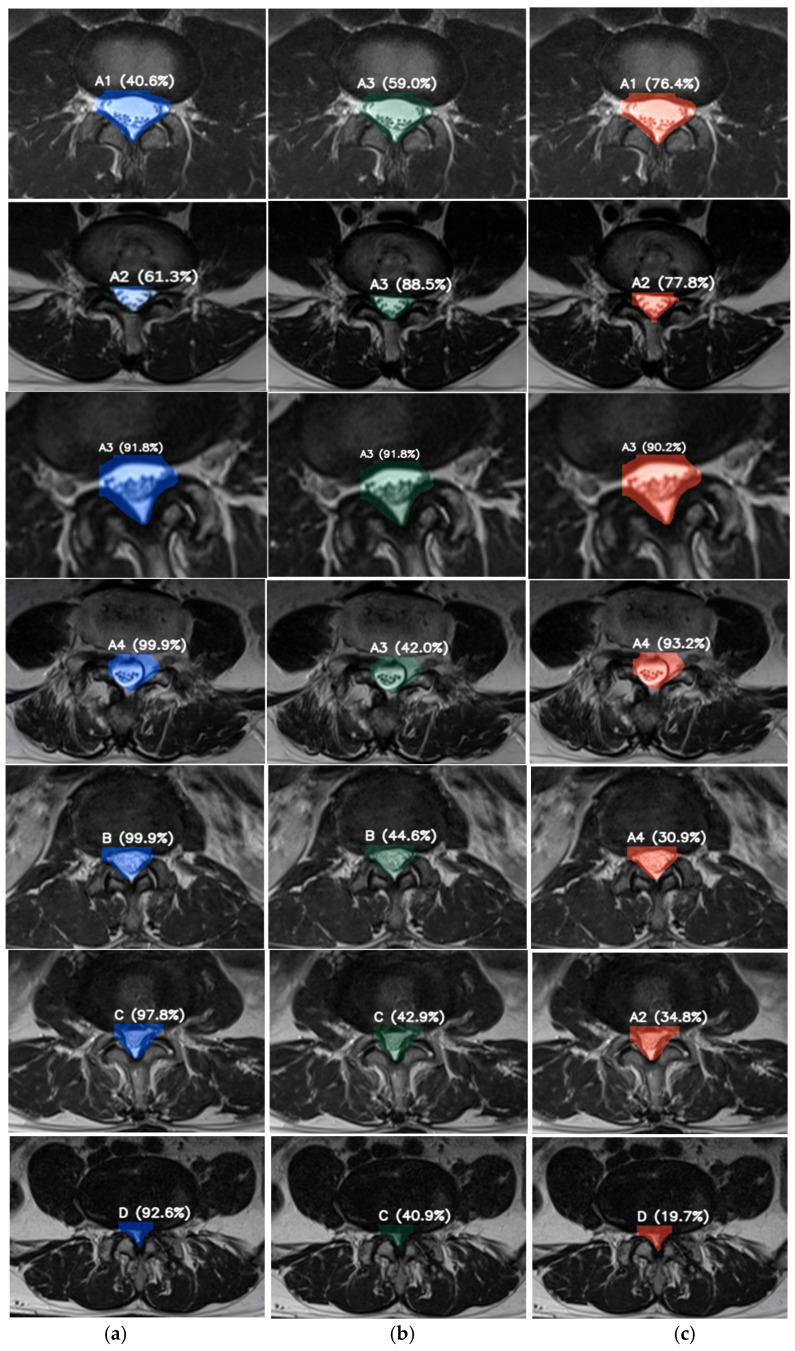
Example predictions using VGG19-based deep features across three machine learning classifiers: (a) Logistic Regression, (b) SVM, and (c) LightGBM. The predicted stenosis grade and associated confidence score are shown on each MRI slice.
